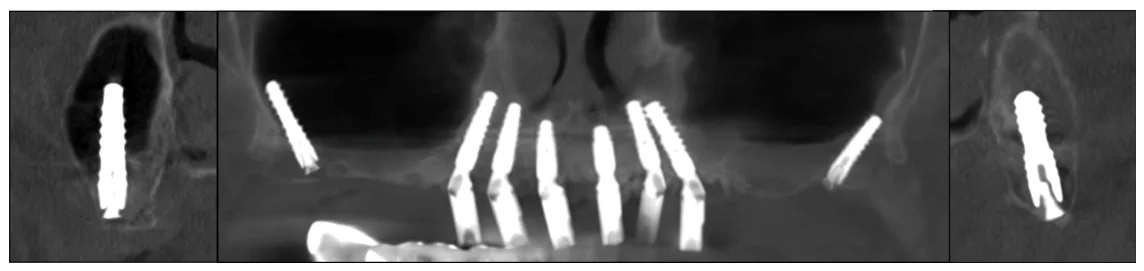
Postoperative CBCT showing displacement of both pterygoid implants into the maxillary sinus.
